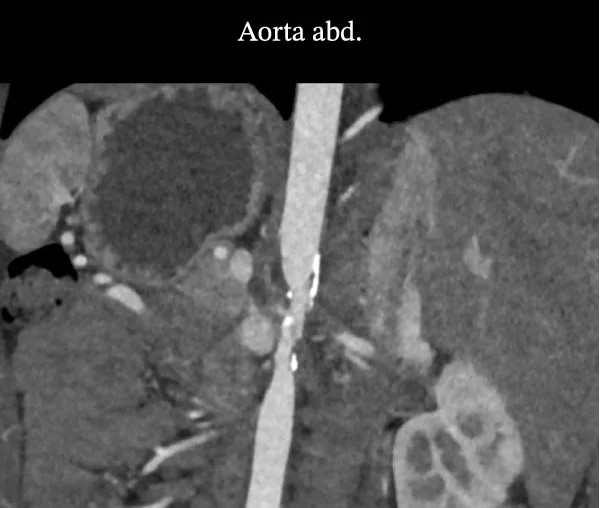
CT angiography, coronary (posterior–anterior) view—severe narrowing of the aorta in the juxtarenal segment.
